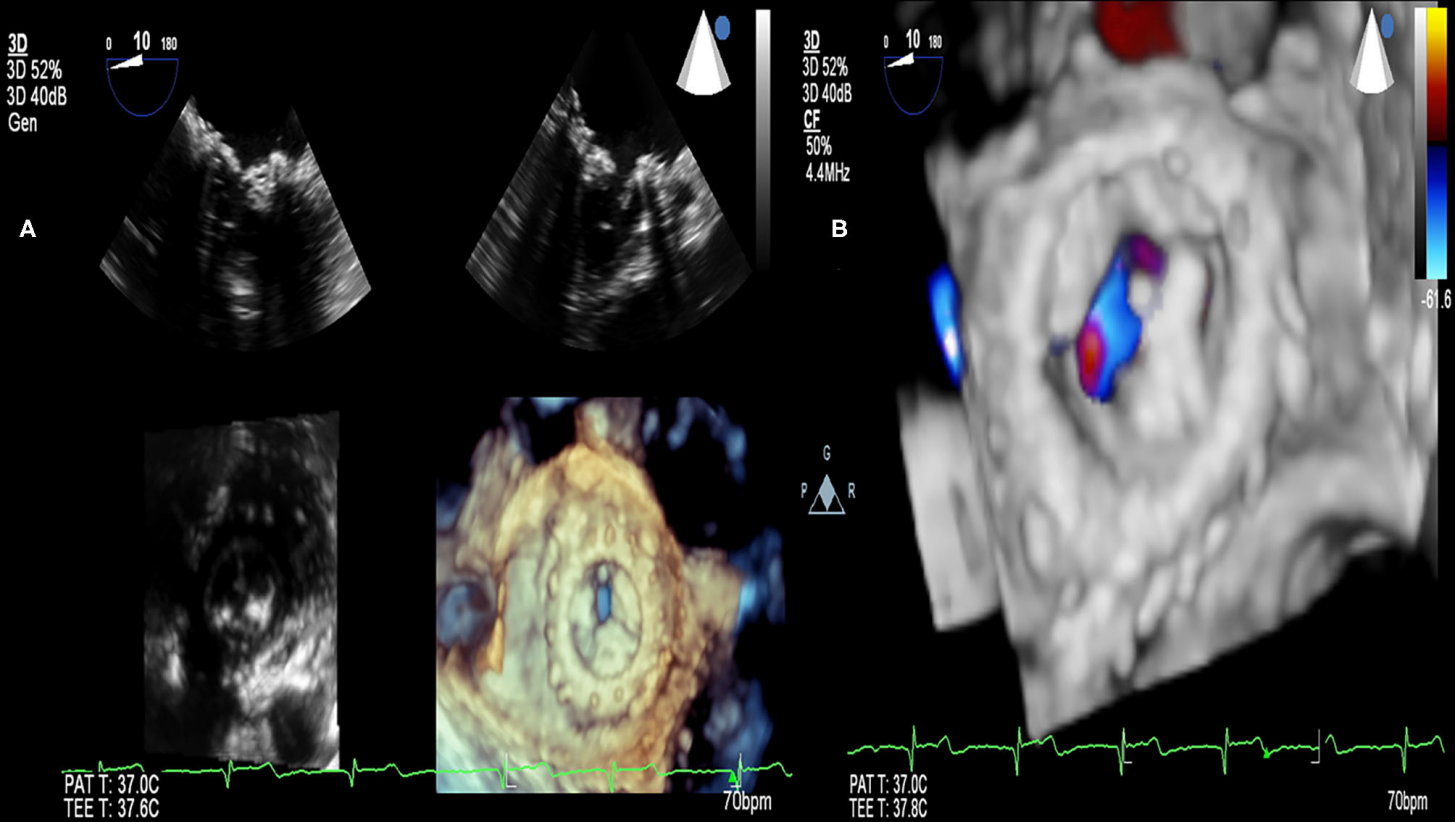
RHD (mitral valve stenosis, stenotic bioprosthesis). (A) 3D transesophageal echocardiography. Demonstration of mitral valve bioprosthesis stenosis (comparison with 2D transesophageal echocardiography). (B) 3D transesophageal echocardiography. Demonstration of mitral valve bioprosthesis stenosis (color Doppler).
